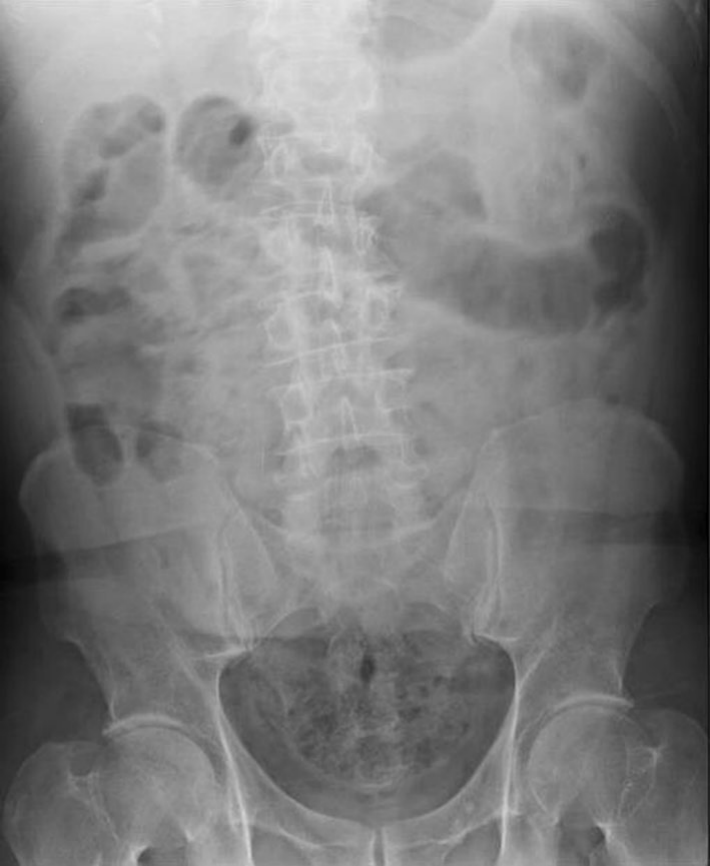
Abdominal X-ray showing diffuse coprostasis and small bowel distension, with no abdominal free air
